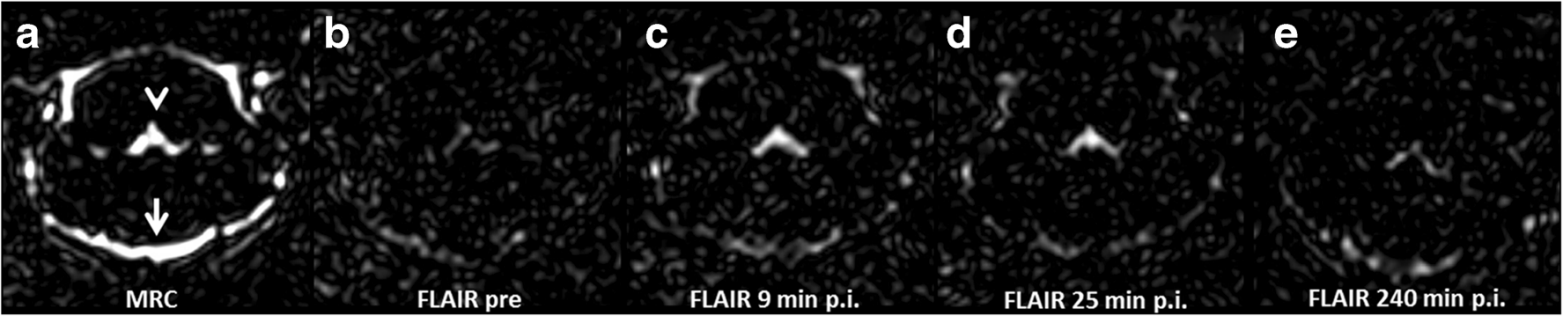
Representative images. The CSF spaces were visualised by MR-cisternography (MRC), for example the fourth ventricle (arrowhead) and the subarachnoid space (arrow) (a). In the fluid-attenuated (FLAIR) images before GBCA injection the respective CSF signal is almost completely attenuated (b). After GBCA administration a clear signal enhancement of the CSF spaces was found in the FLAIR images up to 240 min post injection (p.i.) (c–e)
